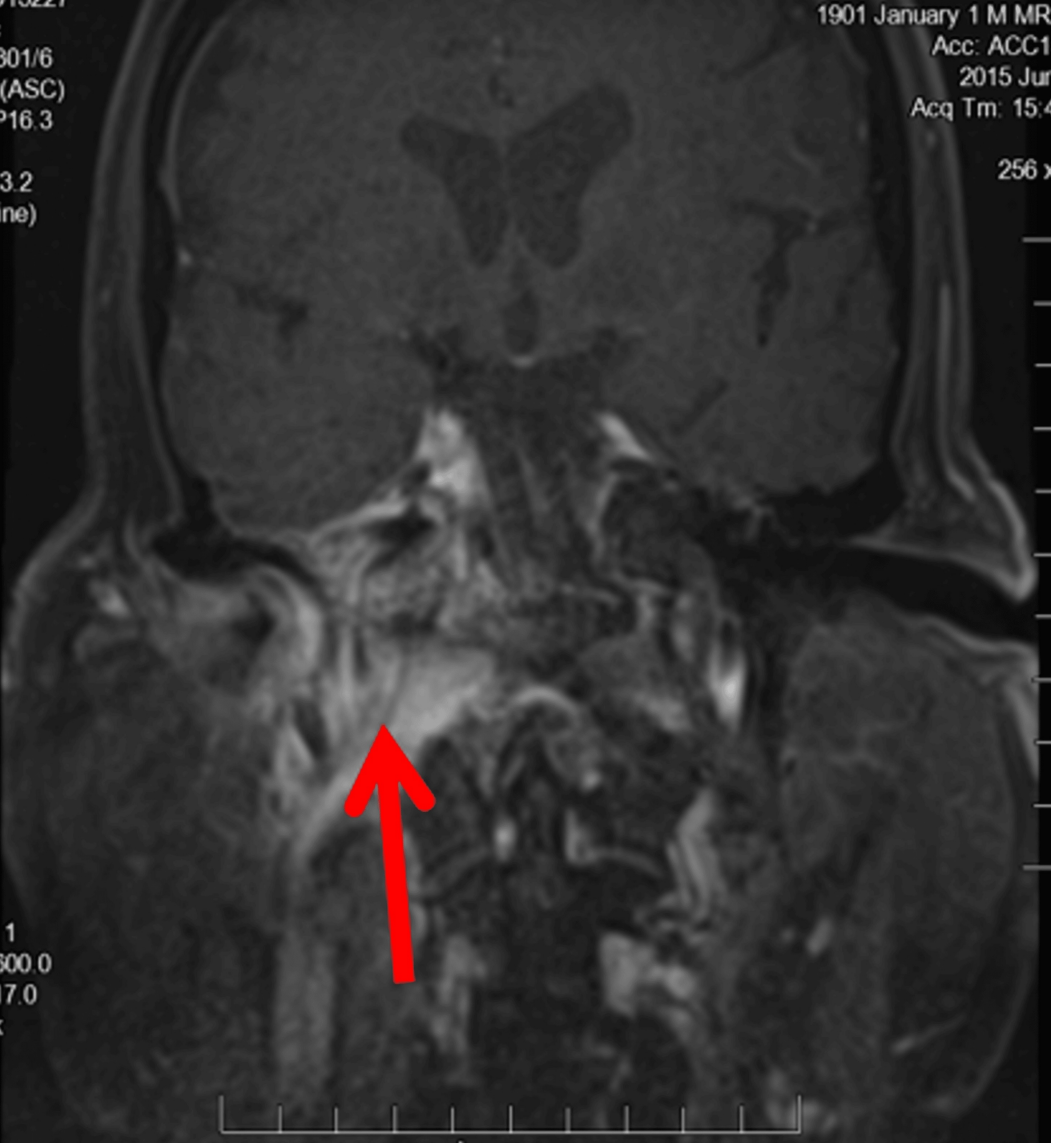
T1-weighted image showing enhancing soft tissue and inflammation near the petrous temporal bone Red Arrow: Enhancing soft tissue and inflammation
